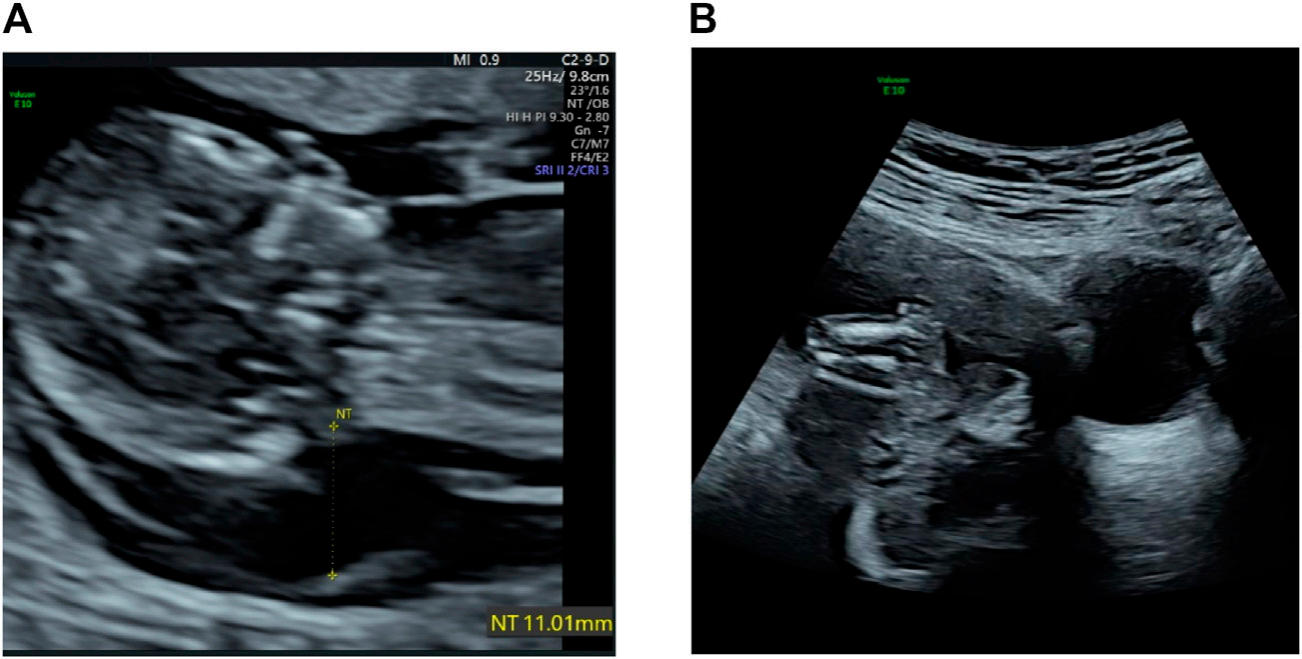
(A) Transabdominal ultrasound at 12 weeks shows a large cystic hygroma. (B) Flexion contracture of lower limb and edema.
